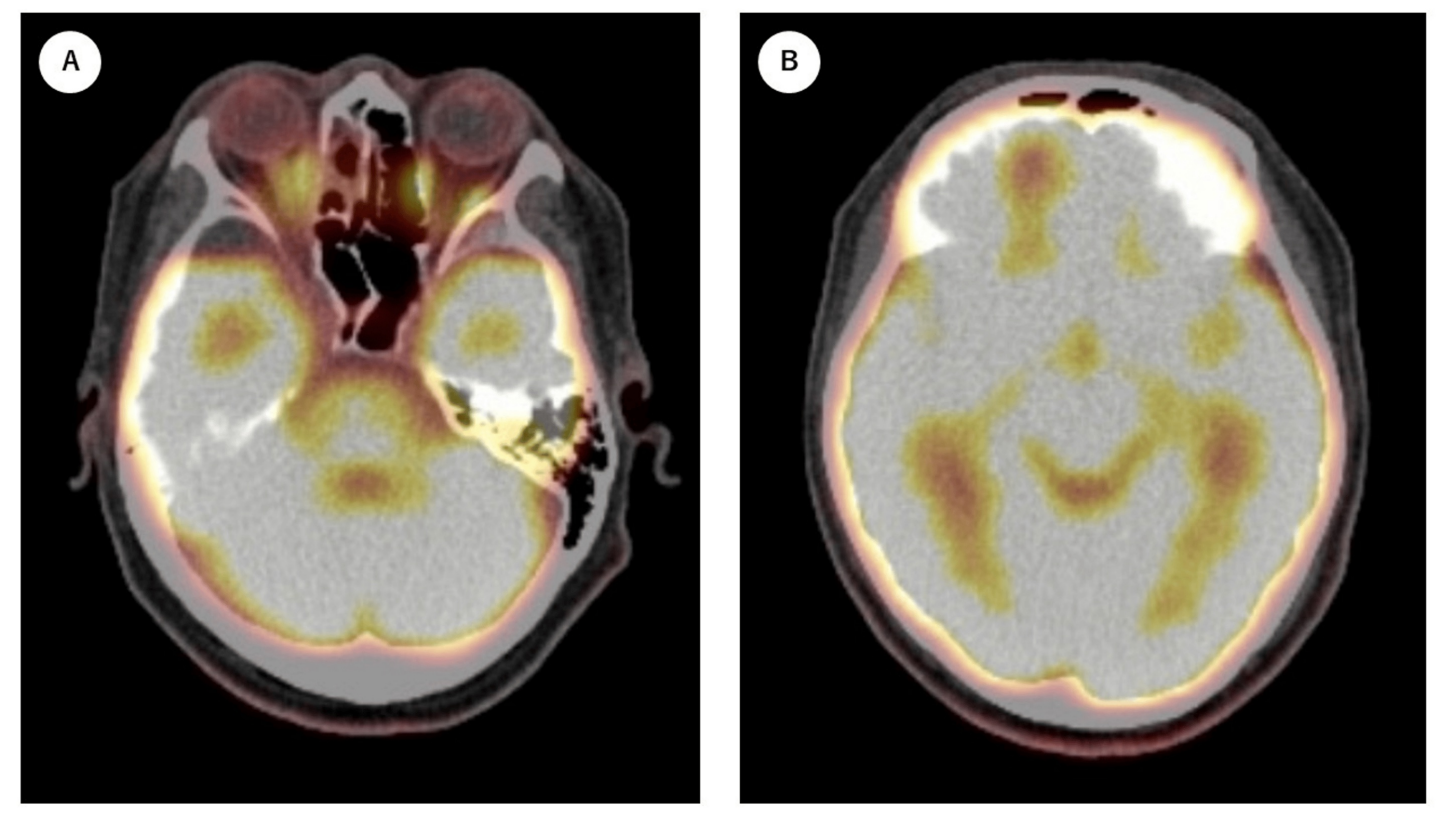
FDG PET imaging six months after completion of treatment. A. FDG accumulation in the right paranasal lesion has disappeared. B. The area surrounding the region of decreased FDG uptake was difficult to evaluate due to physiological accumulation in the brain. FDG: fluorodeoxyglucose; PET: positron emission tomography
